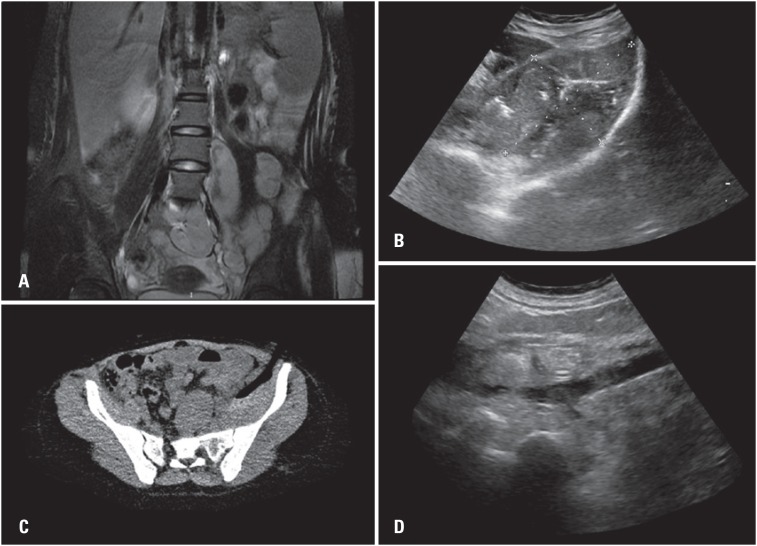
Lumbar spine magnetic resonance imaging, T2-weighted. (A) demonstrates elongated fluid collection in the left psoas. Percutaneous computed tomography-guided drainage was performed (B), with adequate positioning of a Sump (C), Ultrasound control showing important reduction in volume of collection (D)
